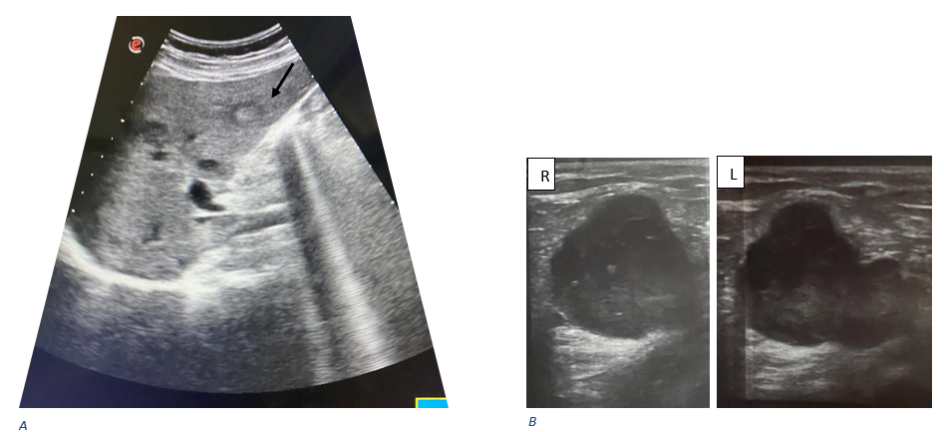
Abdominal ultrasound showing (A) hepatic lesions (black arrow) and (B) bilateral inguinal lymph nodes, right and left sides.
